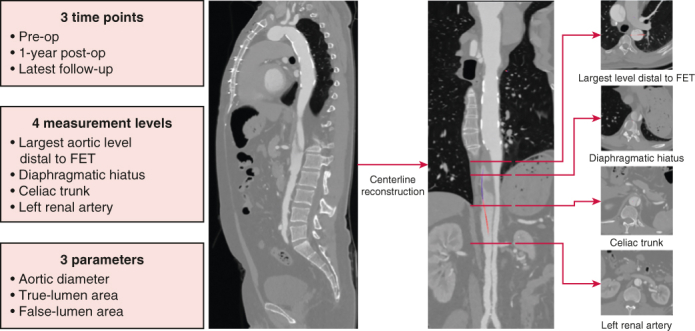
Collecting the morphological and hemodynamic characteristics from CT images.
